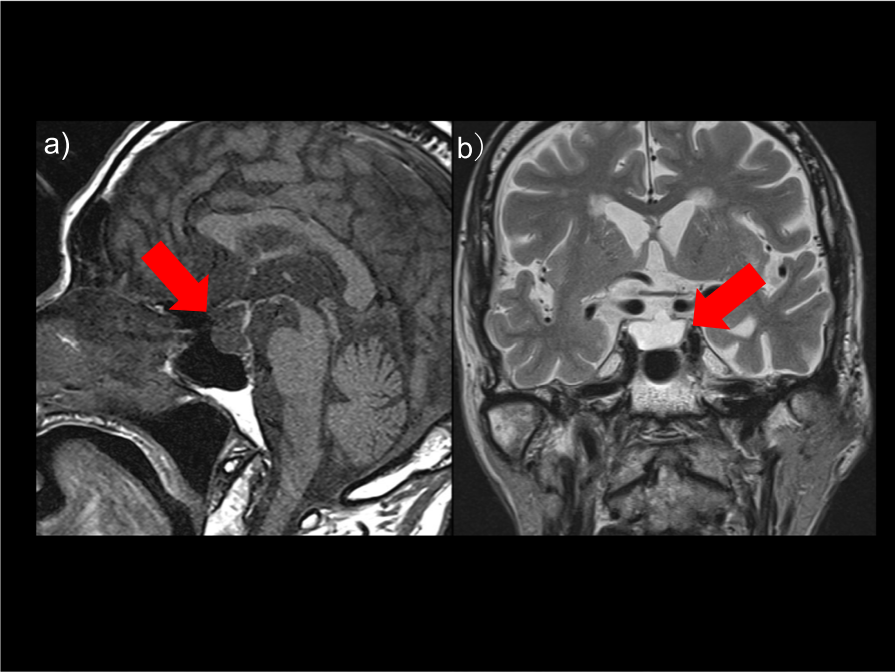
Contrast-enhanced pituitary magnetic resonance imaging. a T1-weighted image – sagittal section; (b) T2-weighted image – coronal section shows empty sella
